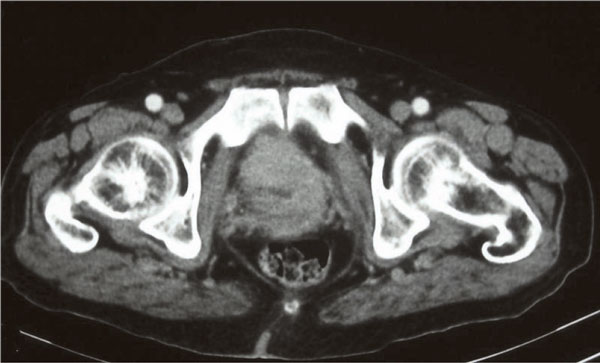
CT scan of the pelvis shows the tumoral process infiltrated the base of the prostate gland.
